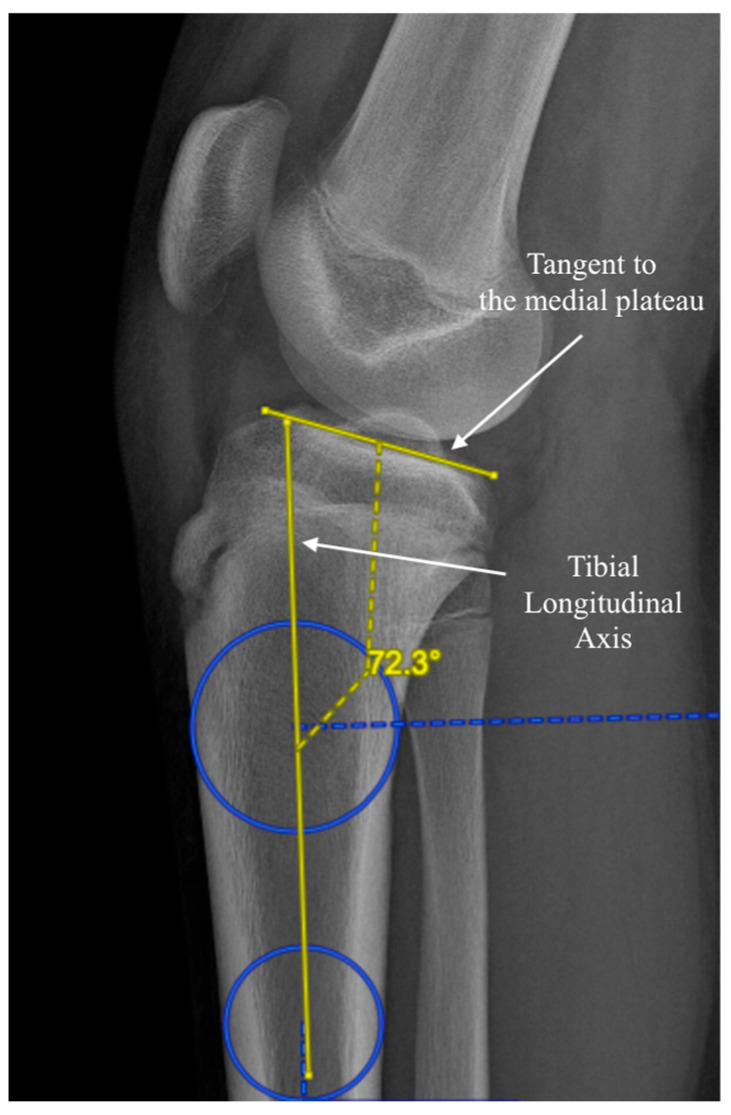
Lateral Knee Radiograph demonstrating the 2-circle method in measuring the medial posterior tibial slope (MPTS). The tibial anatomical axis is defined by two circles placed along the tibial shaft. The posterior tibial slope is calculated as 90° minus the angle (α) between this axis and the tangent to the medial tibial plateau.
